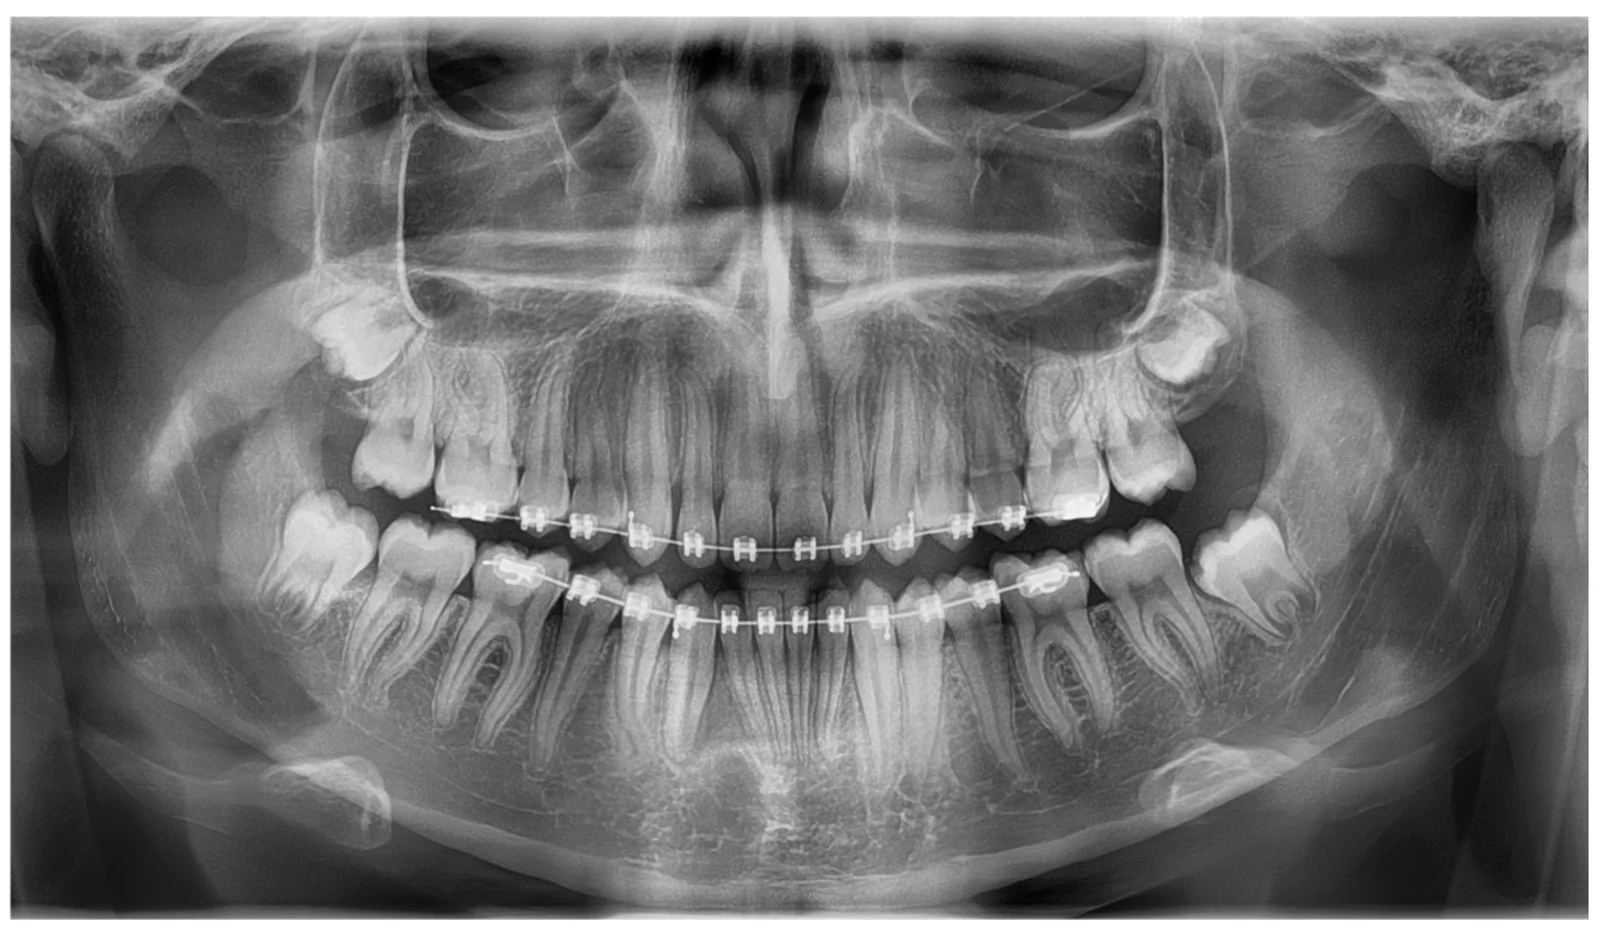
Pre-final panoramic radiograph.
